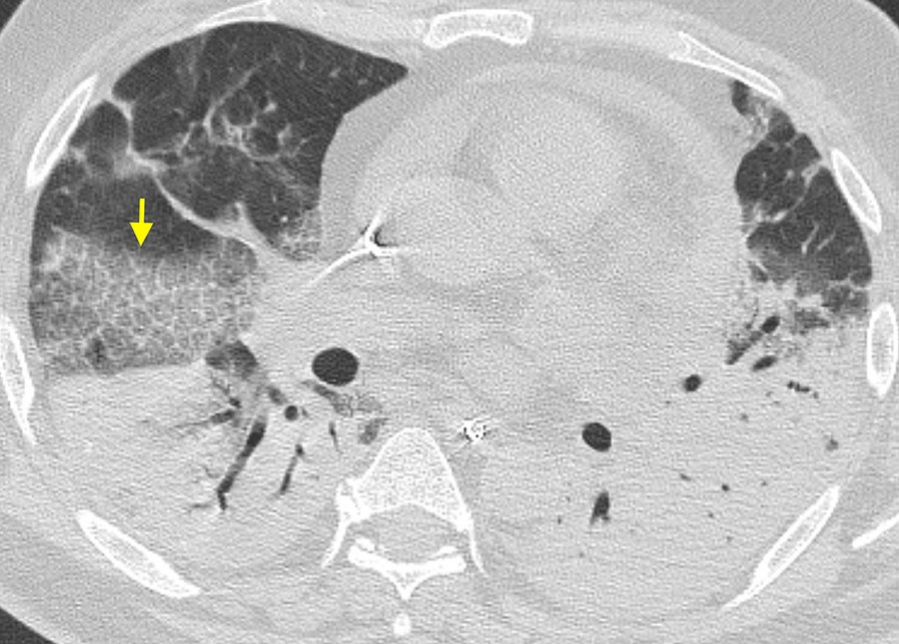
Chest CT imaging appearance of severe COVID-19 pneumonia and acute respiratory distress syndrome (ARDS). High-resolution CT image of a COVID-19 patient admitted to intensive care unit shows bilateral areas of consolidation predominantly affecting the dependent areas of both lungs, along with ground-glass opacity (GGO) with “crazy-paving” pattern in the right middle lobe (arrow)
